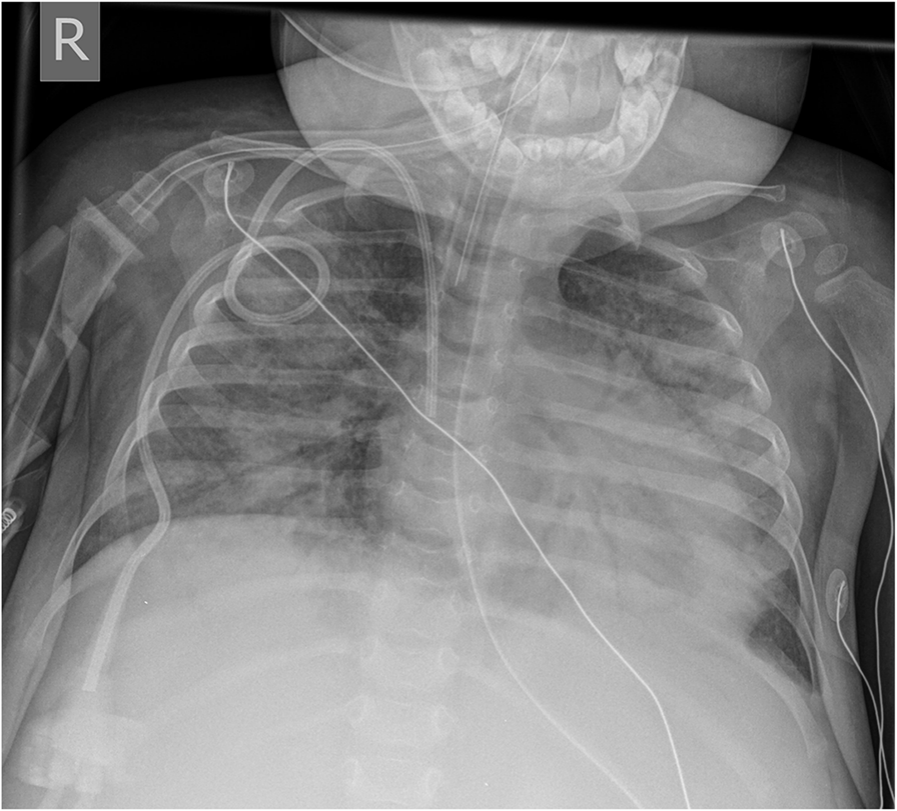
Supine portable chest radiograph for a 15-month-old girl with sideroblastic anemia and immunodeficiency, periodic fever and developmental delay (SIFD), 1 month after bone marrow transplant: the radiograph demonstrates diffuse bilateral pulmonary opacities without cardiomegaly. Note the endotracheal tube and the right central venous line. The patient died with acute respiratory distress syndrome due to graft-versus-host disease
